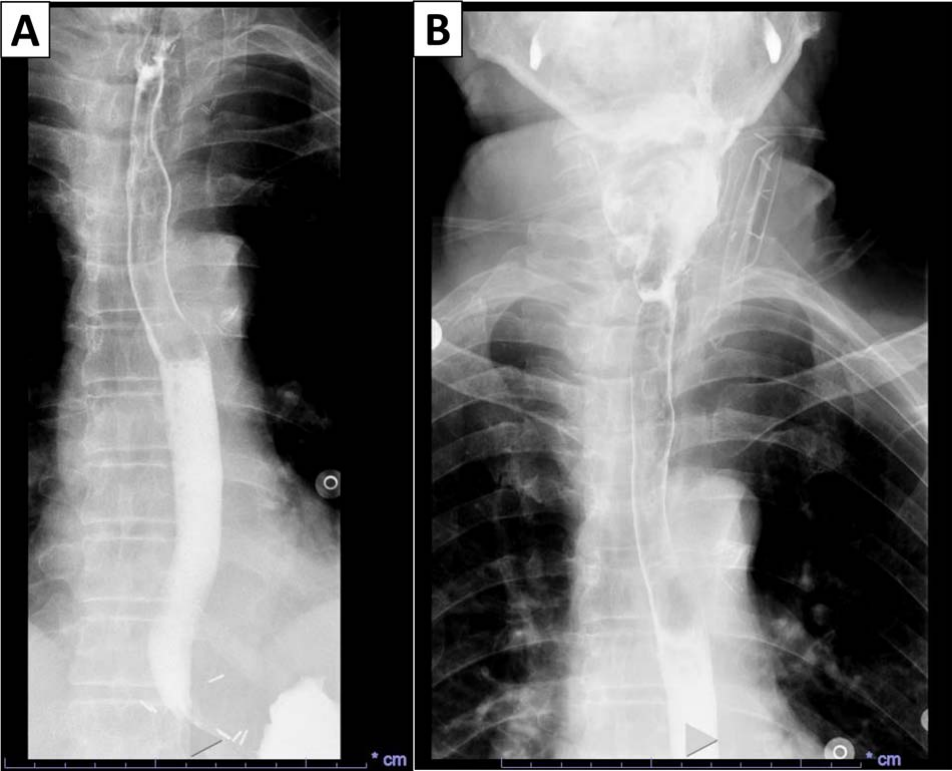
X-ray esophagogram performed postoperatively. No postoperative leak and no residual ZD were seen.
